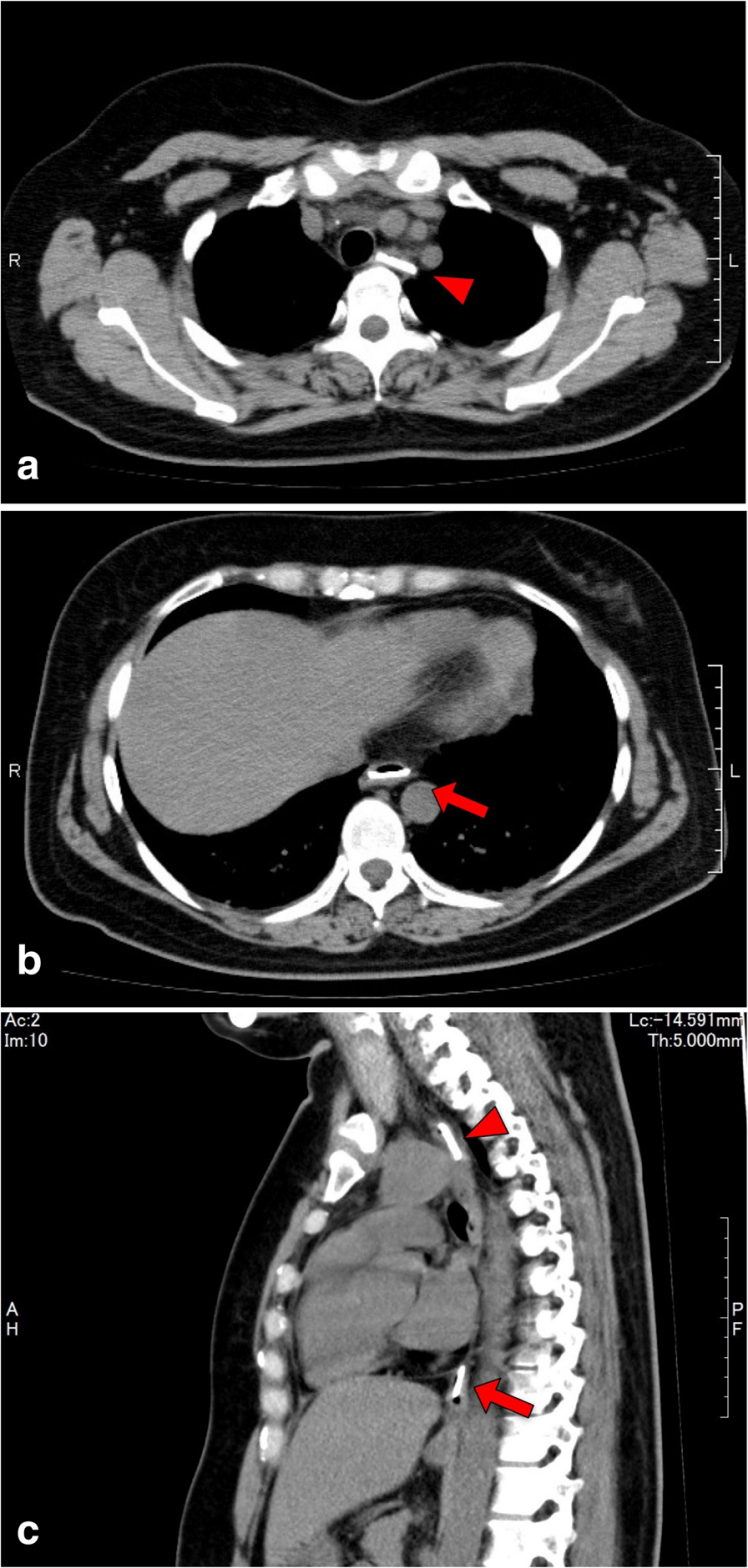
On the initial computed tomography (CT) scan, one coin (*arrowhead*) was found in the middle oesophagus (a) and the other (*arrow*) was in the lower oesophagus (b). In the sagittal CT multiplanar reconstruction image (c), both the coins are observed simultaneously
